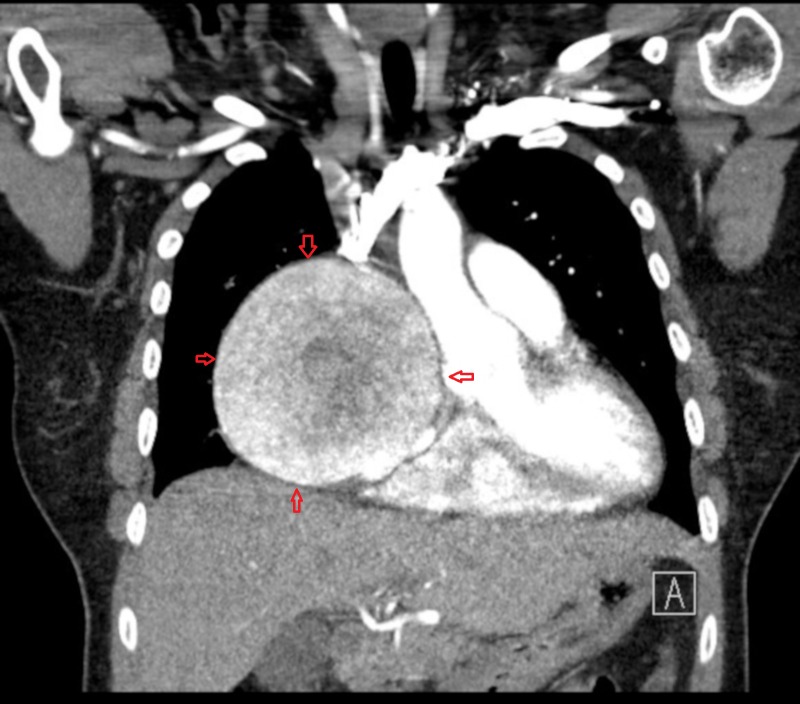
CCTA showing an anomalous vessel arising from the proximal left circumflex artery and giving rise to a 9.7 cm coronary artery aneurysm CCTA: coronary computed tomography angiography
